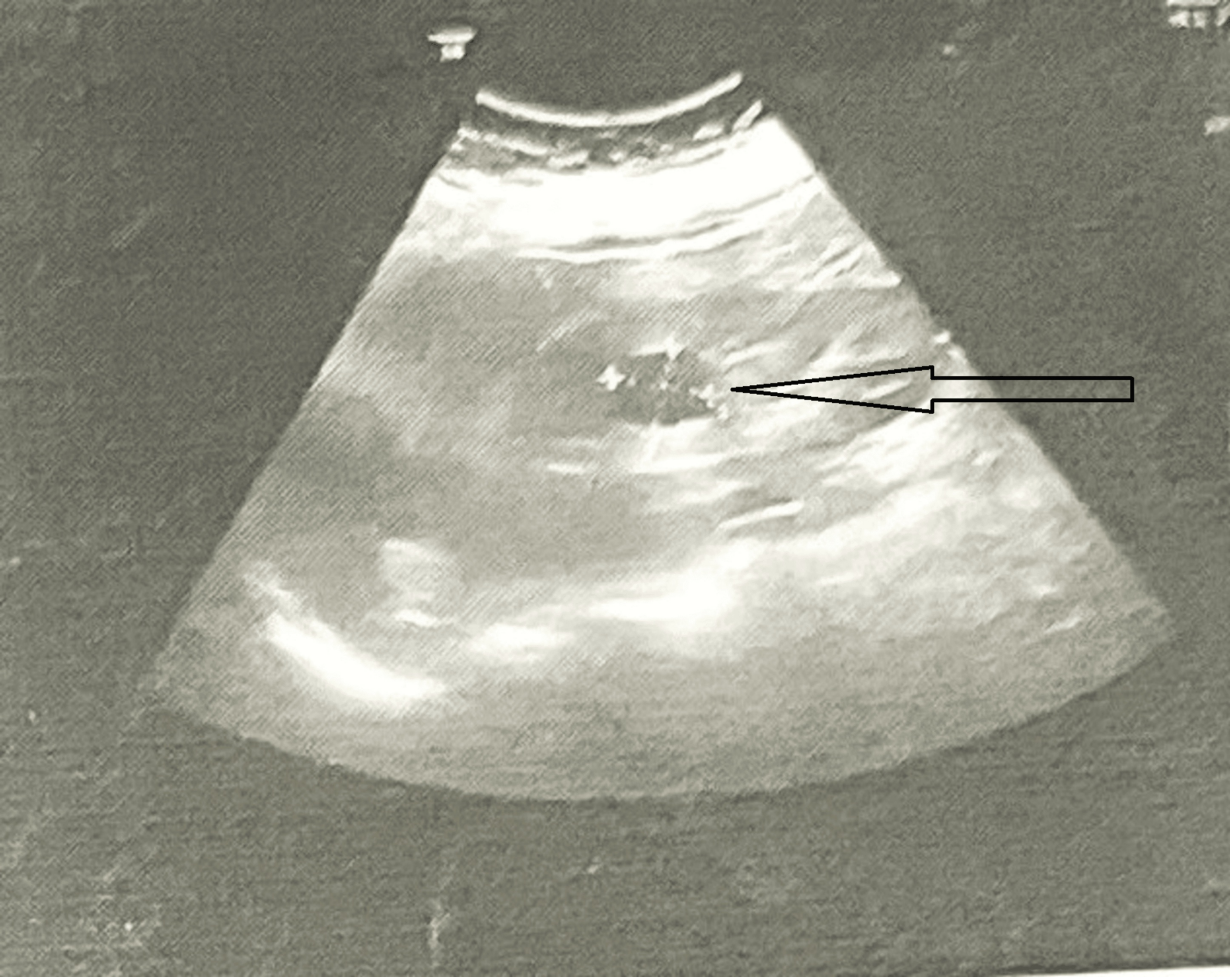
Ultrasound of the abdomen showing a left renal cystic lesion A cystic lesion measuring 23 x 23 mm without internal septations/ echoes was noted in the mid-pole region, suggesting a simple corticomedullary cyst (arrow).
